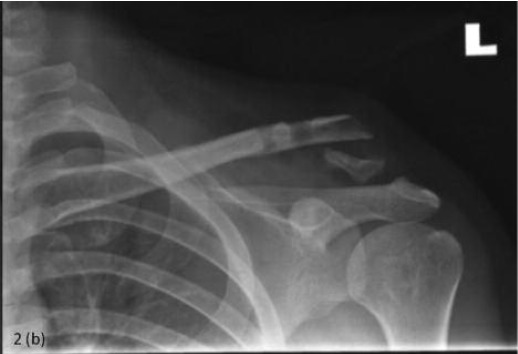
5 months post operative radiograph showing nonunion
